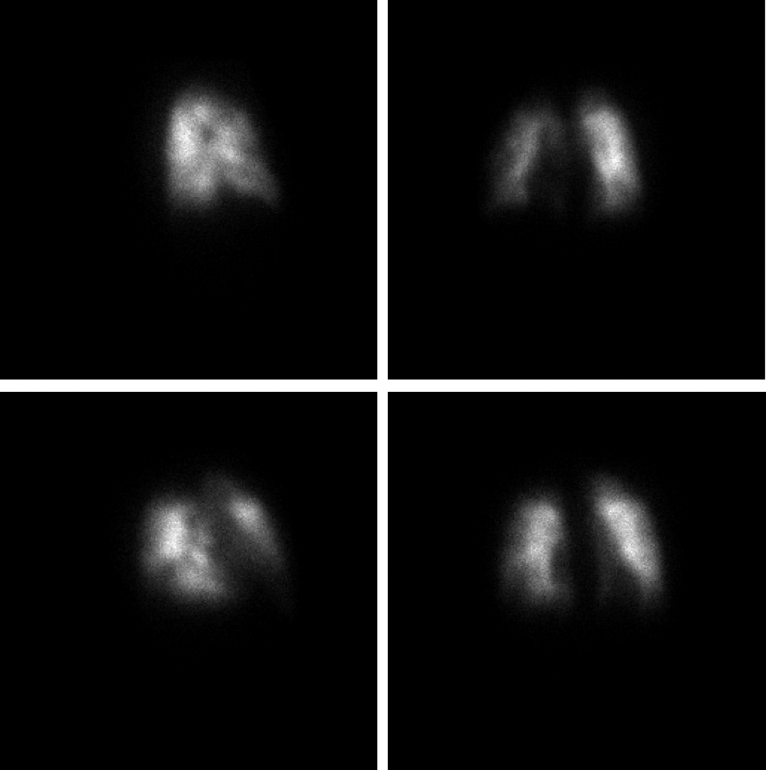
Planar perfusion scintigraphy showing multiple segmental mismatched perfusion deficits.
